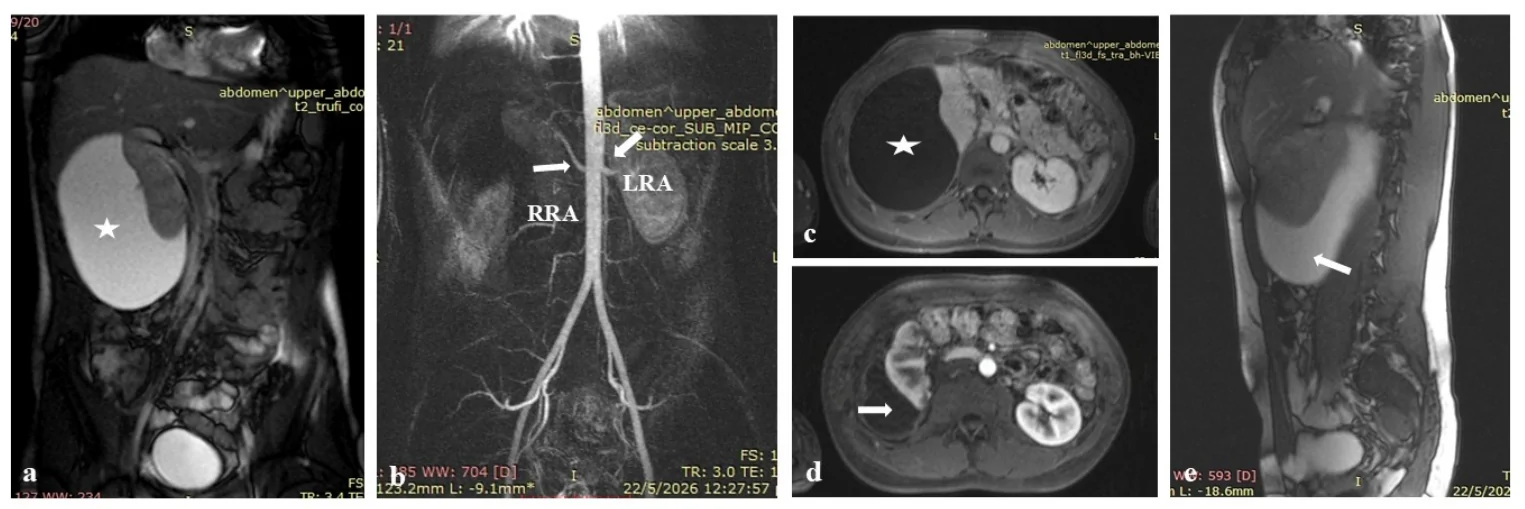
MR imaging of the patient’s upper abdomen/retroperitoneum revealing a significant fluid collection in the perinephric region of the right kidney consistent with a chronic liquefied hematoma (a–e). The collection severely compresses the parenchyma of the right kidney. (a,e) Sagittal magnetic resonance (MR) imaging planes of the upper abdomen/retroperitoneum before (a) and after (e) percutaneous drainage with significant decrease in the size of the collection post-drainage (white star and white arrow respectively) (b). Renal artery MR angiography (MRA) providing imaging of the aorta and renal arteries (arrows) without any significant stenoses of the renal artery stumps. Arterial dissection and active bleeding were ruled out (a,e). (c,d) Corresponding tangential MR imaging planes of the kidneys after nephrostomy catheter insertion and drainage of 1700 mL of the serous collection underneath the renal (Gerota) fascia ((c): before drainage indicated with a star, (d): after drainage indicated with an arrow). RRA: Right Renal Artery; LRA: Left Renal Artery.
